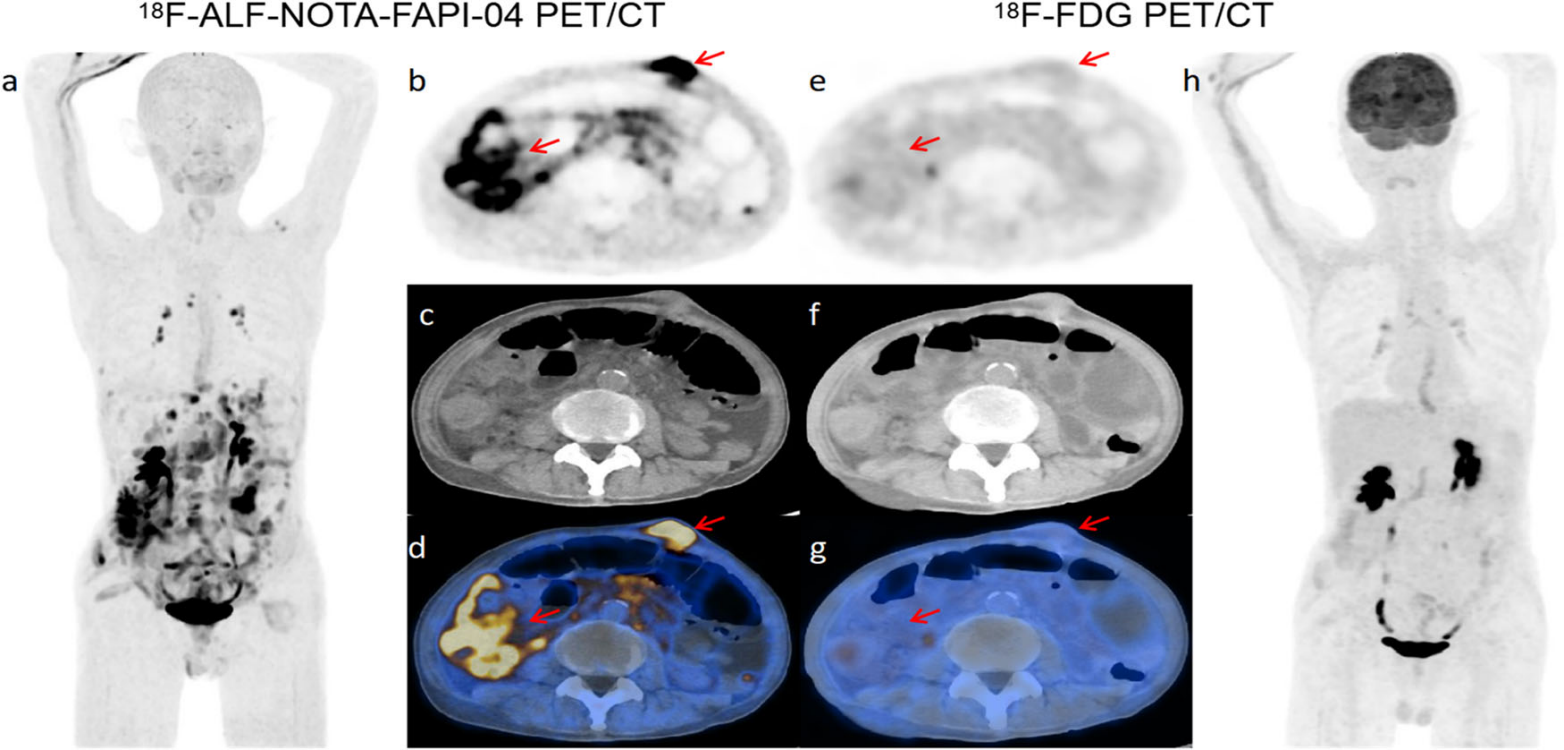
A 57-year-old male patient with poorly differentiated adenocarcinoma presented with abdominal distention and pain after postoperative chemotherapy. [^18^F]ALF-NOTA-FAPI-04 PET/CT (a–d) revealed diffuse high uptake in the peritoneum (SUVmax = 13.3) and the left anterior lower abdominal wall (SUVmax = 20.2), whereas [^18^F]FDG PET/CT (e–h) demonstrated mild uptake in the peritoneum (SUVmax = 2.1) and the left anterior abdominal wall (SUVmax = 2.2)
